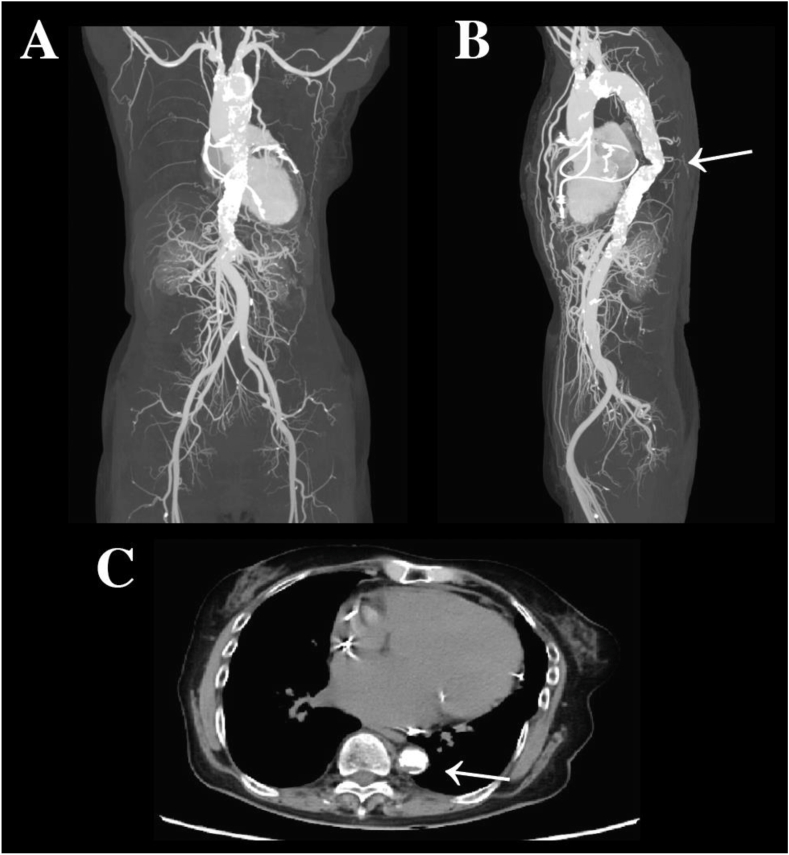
Preoperative computed tomography image of the aorta (A) Frontal view. (B) Left lateral view. (C) Axial plane. The region indicated by the arrow in (B) and (C) depicts the stenotic segment of the aorta characterized by advanced calcification.
